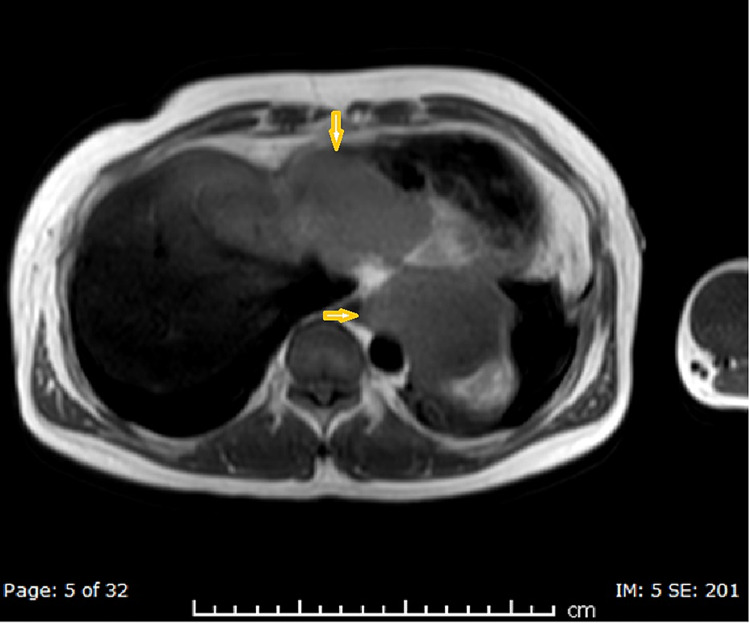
MRI T2-weighted axial image showing a pericardial mass lesion (arrowheads)
